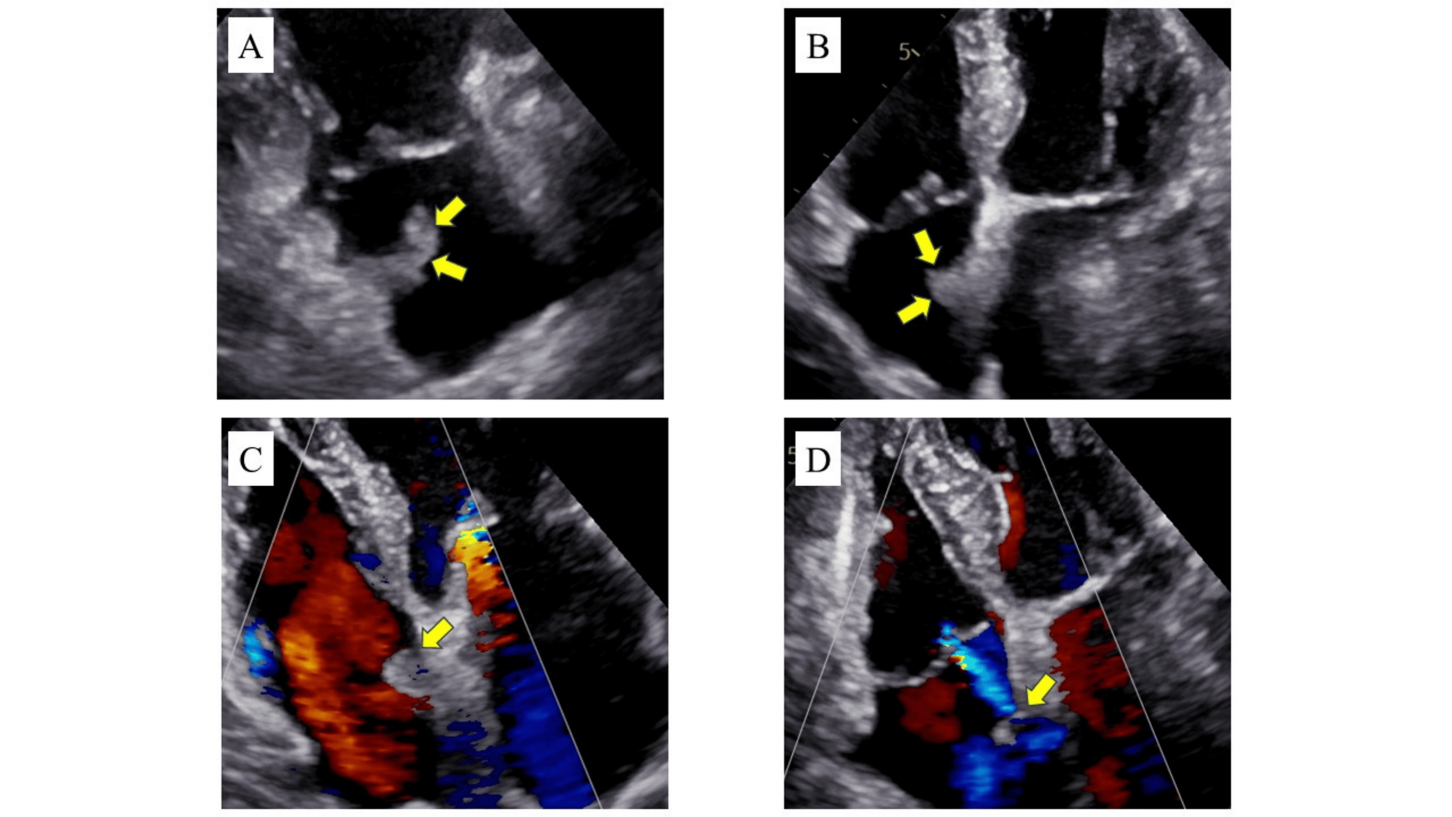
Preoperative TTE (A-D) TTE revealed that the tumor (yellow arrows), measuring 13 × 9 mm, was attached to the right atrial septum, highly mobile, well-defined with smooth edges, and exhibited homogeneous internal echogenicity without blood flow within the tumor. No interference with the tricuspid valve which could cause blood flow acceleration or severe regurgitation was detected. No other significant valvular diseases were noted. TTE: transthoracic echocardiography.
